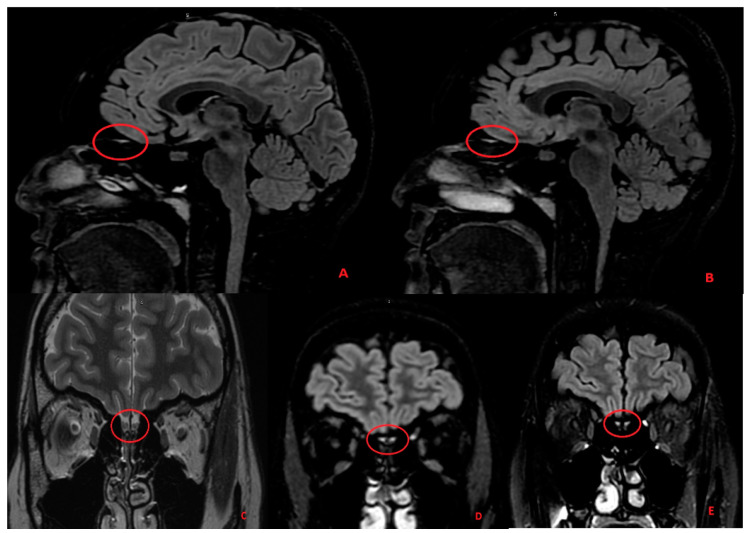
In the images above, we can see the hyperintensity of both olfactory bulbs (Panel A,B, sagittal FLAIR images) and olfactory tracts (Panel C coronal T2 FRFSE image; Panel D,E coronal FLAIR images) in the sequences obtained in the first magnetic resonance (MR) executed on the patient. In the coronal T2 FRFSE sequence (Panel C), we can also see that both olfactory tracts are swollen and hyperintense in their central portion, and that is even more evident for the right olfactory tract.
